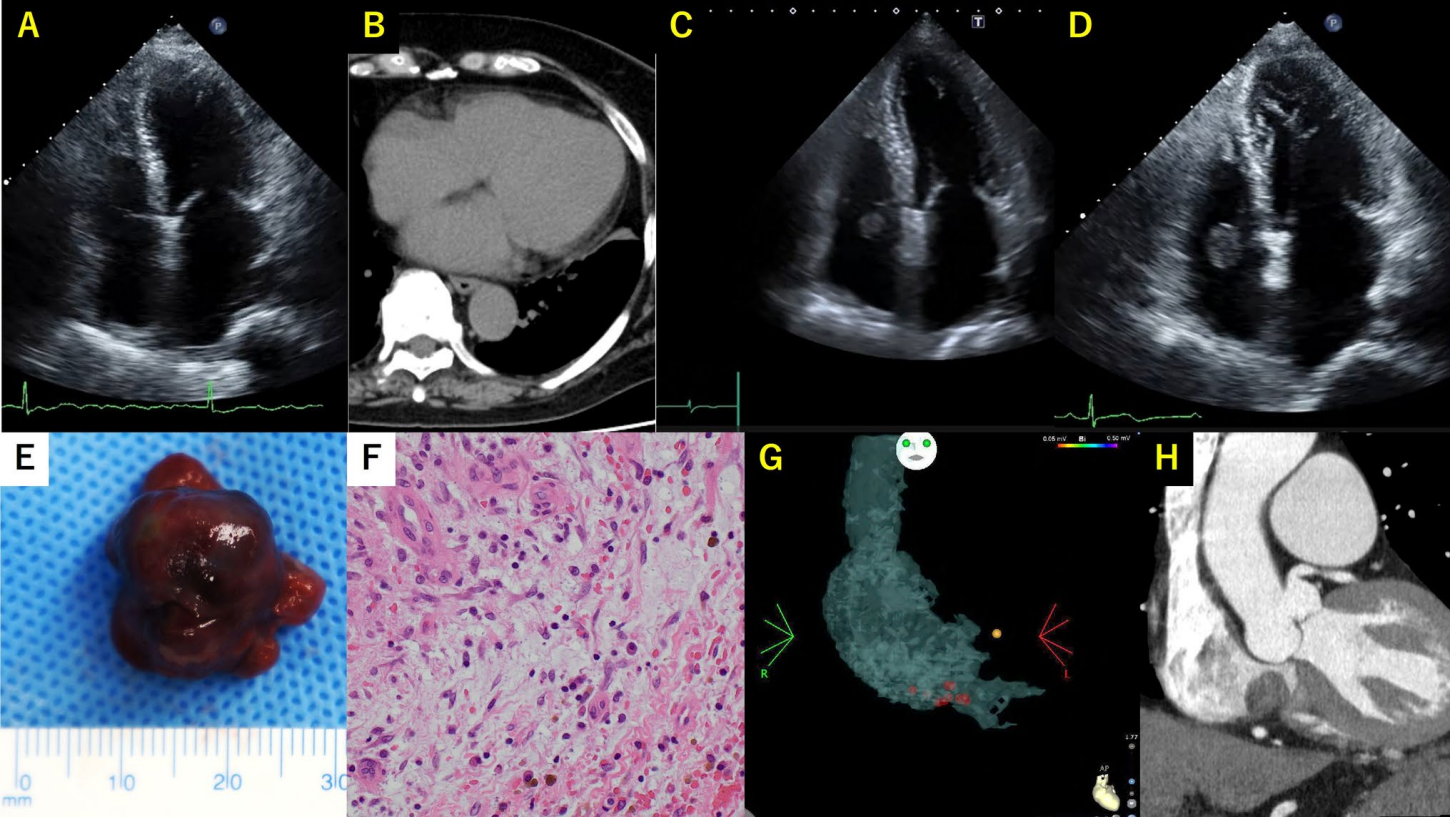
Panel A. Transthoracic echocardiogram (TTE) apical four-chamber view, performed before radiofrequency ablation (RFA), showed no mass in the right atrium. Panel B. Computed tomography (CT) horizontal section, performed before RFA, showed no mass in the right atrium. Panel C. TTE apical four-chamber view, performed one year after RFA, revealed a 17 mm × 11 mm mobile mass in the right atrium. Panel D. TTE apical four-chamber image performed two years after RFA, revealed a 21 mm × 20 mm mobile mass in the right atrium. Panel E. Surgically removed 20 mm x 20 mm sized mass. Panel F. Pathological findings of the excised mass (strongly magnified image). Panel G and H. Ablation sight at the cavotricuspid isthmus (G) compared with preoperative cardiac contrast-enhanced CT coronal section (H)
